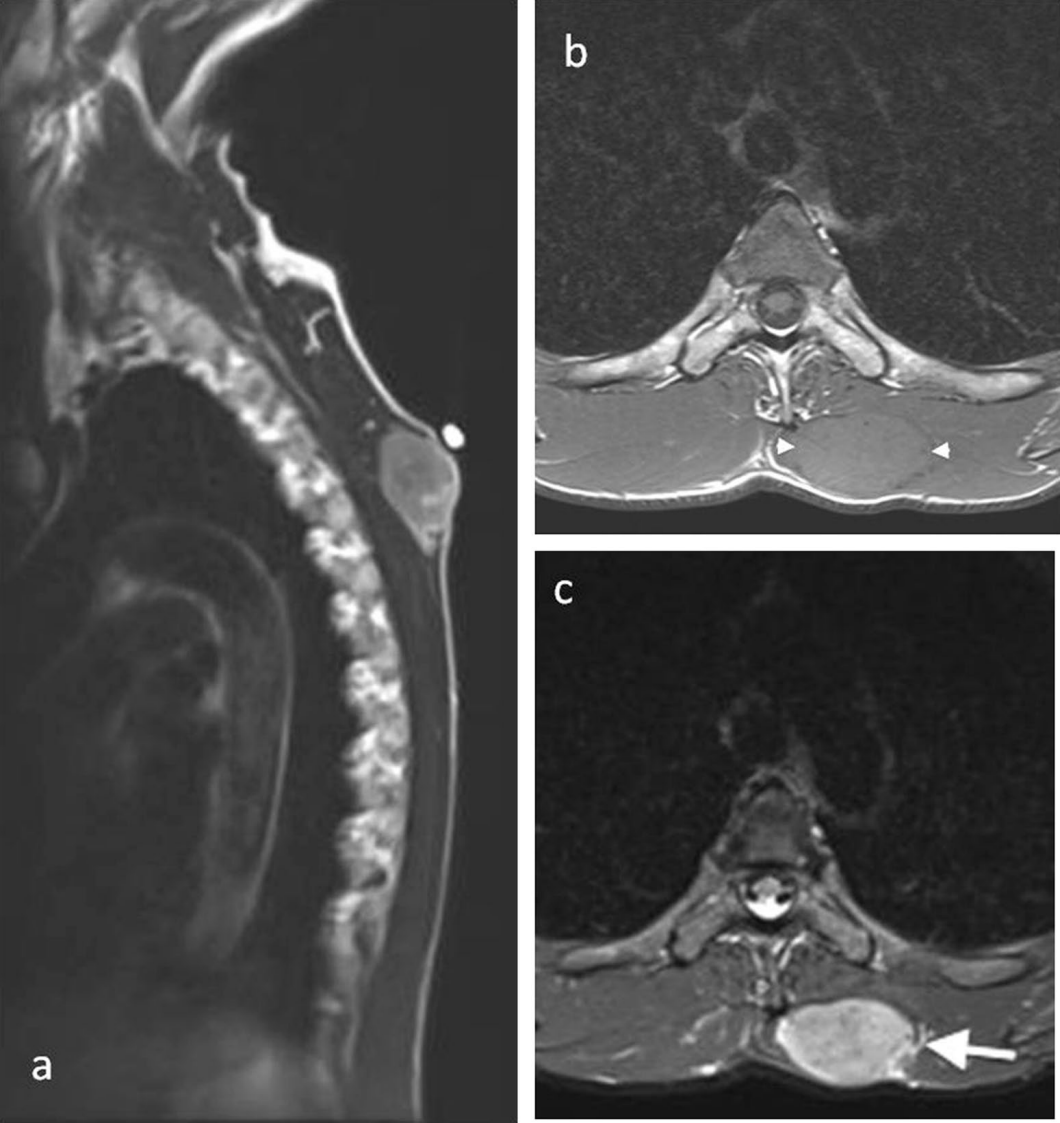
Sagittal T2 (a), axial T1 (b) and axial T2 fat saturated (c) MR images demonstrate a solid well defined soft tissue mass in the medial left trapezius muscle which returns isointense T1-W (between *arrow heads*) and heterogeneous predominantly high T2-W signal relative to skeletal muscle. There is mass effect on the deeper paravertebral muscles and peripheral feeding vessels along the lateral aspect of the lesion (*arrow*)
